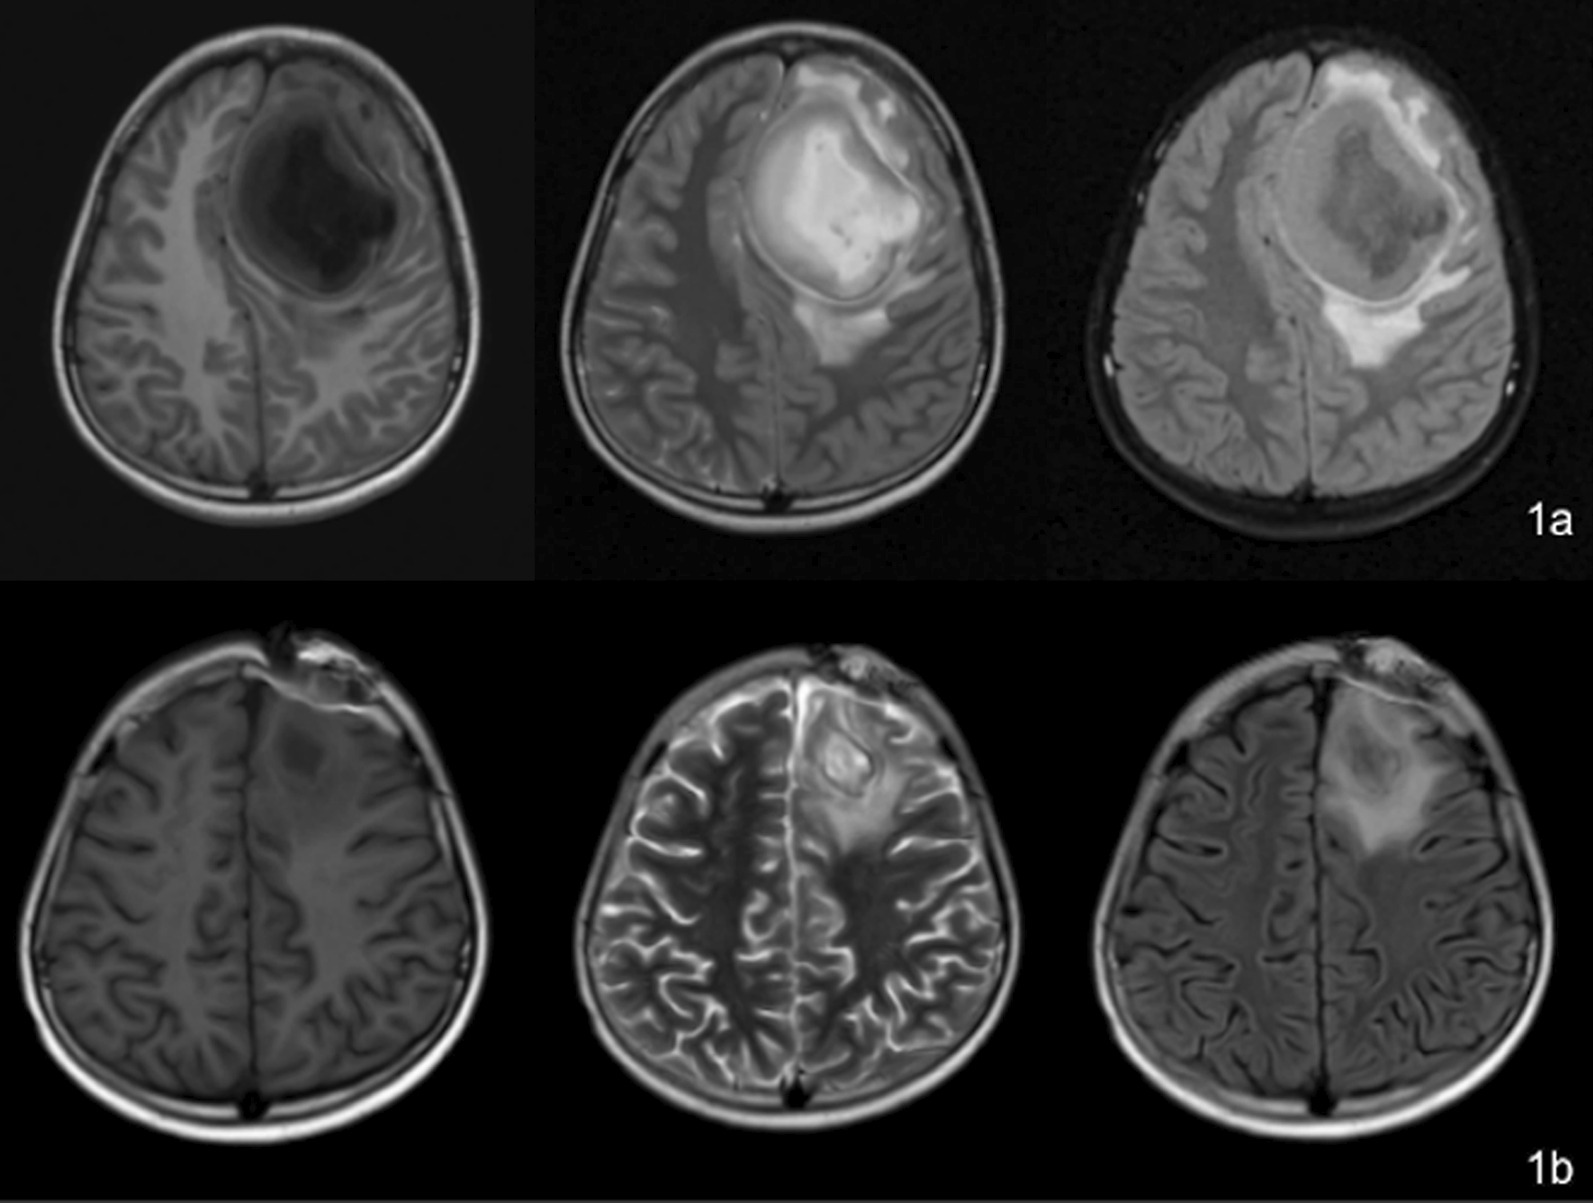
a Head MRI before the brain abscess showed cystic occupation of the left frontal lobe. b Head MRI after excision of brain abscess showed the occupying effect in the left frontal lobe was significantly reduced
